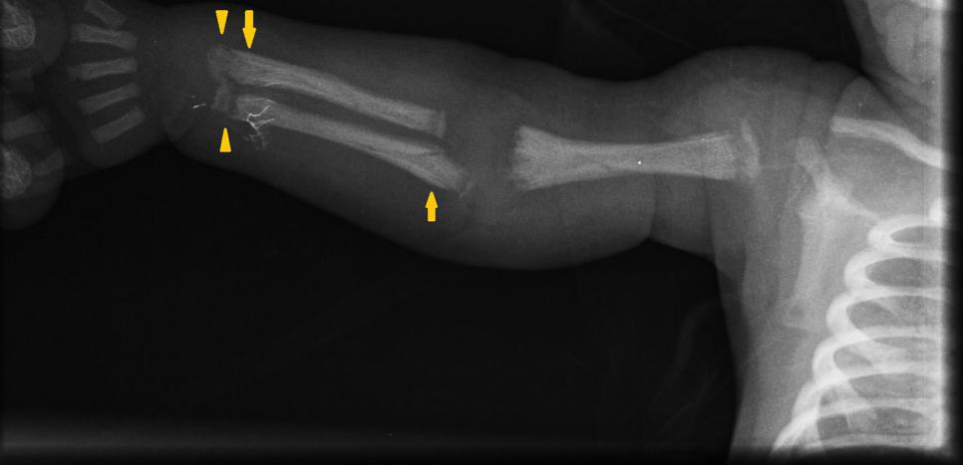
Radiographic evidence of periostitis and metaphyseal bands in upper right extremity long bones.
